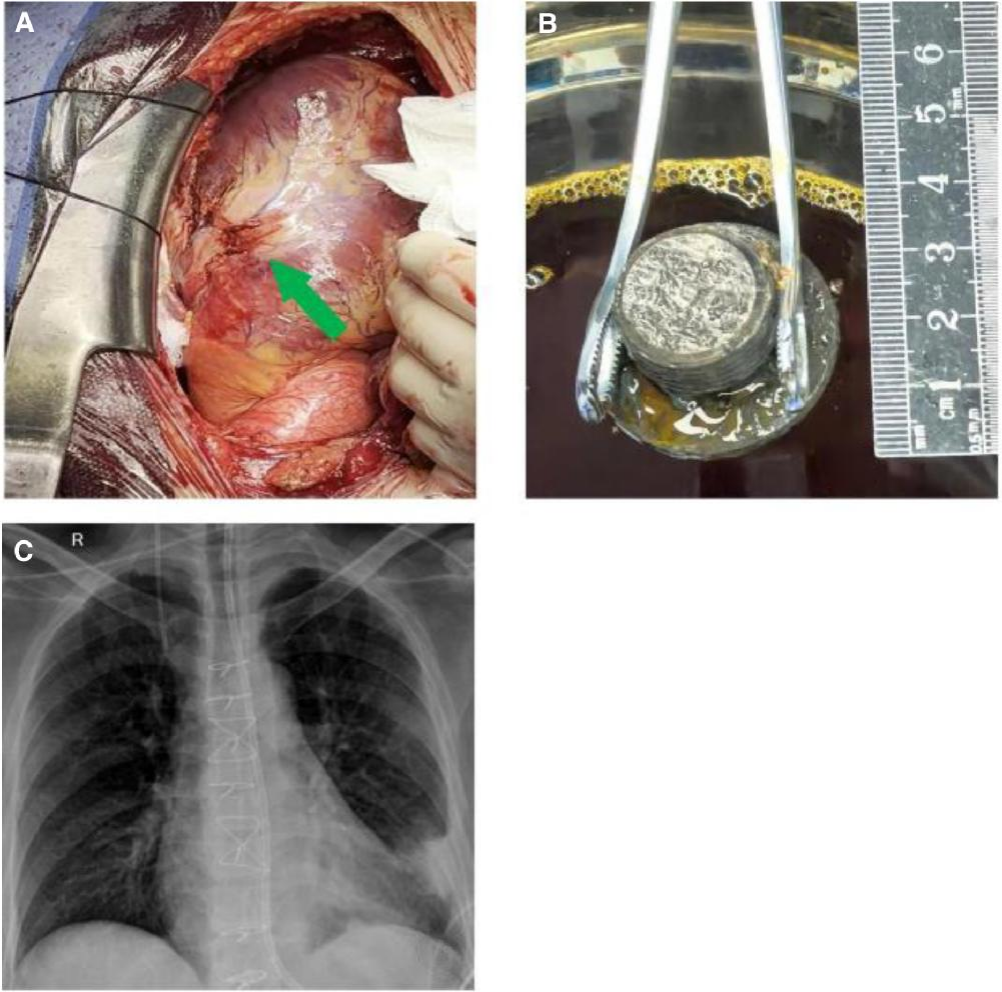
(*A*) The solid arrow shows a contusion of the left ventricular lateral wall and complete rupture of the left anterior descending artery. (*B*) The screw removed from the thoracic cavity during the surgery. (*C*) The post-surgery X-ray demonstrated an optimal recovery.
